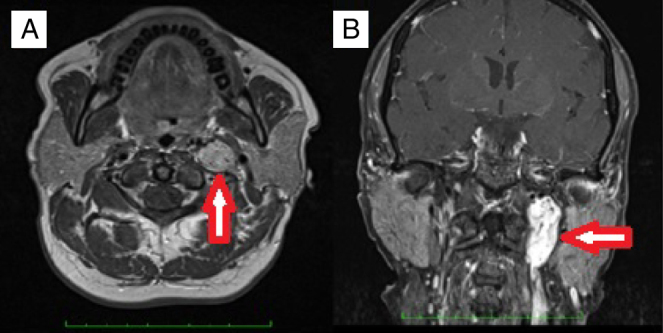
A case of left sided TJP with typical salt and pepper appearance; meaning areas of high and low intensity on MRI. (A) An axial section of T1 weighted sequences. (B) A coronal section of T1 weighted fat suppressed sequences.
